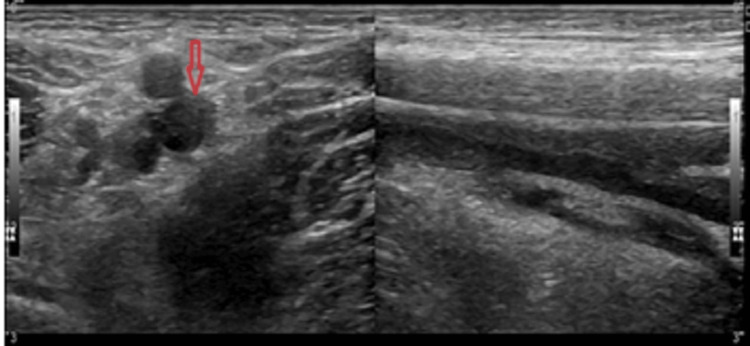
Doppler ultrasound showing thrombosed femoral vein (arrow)
